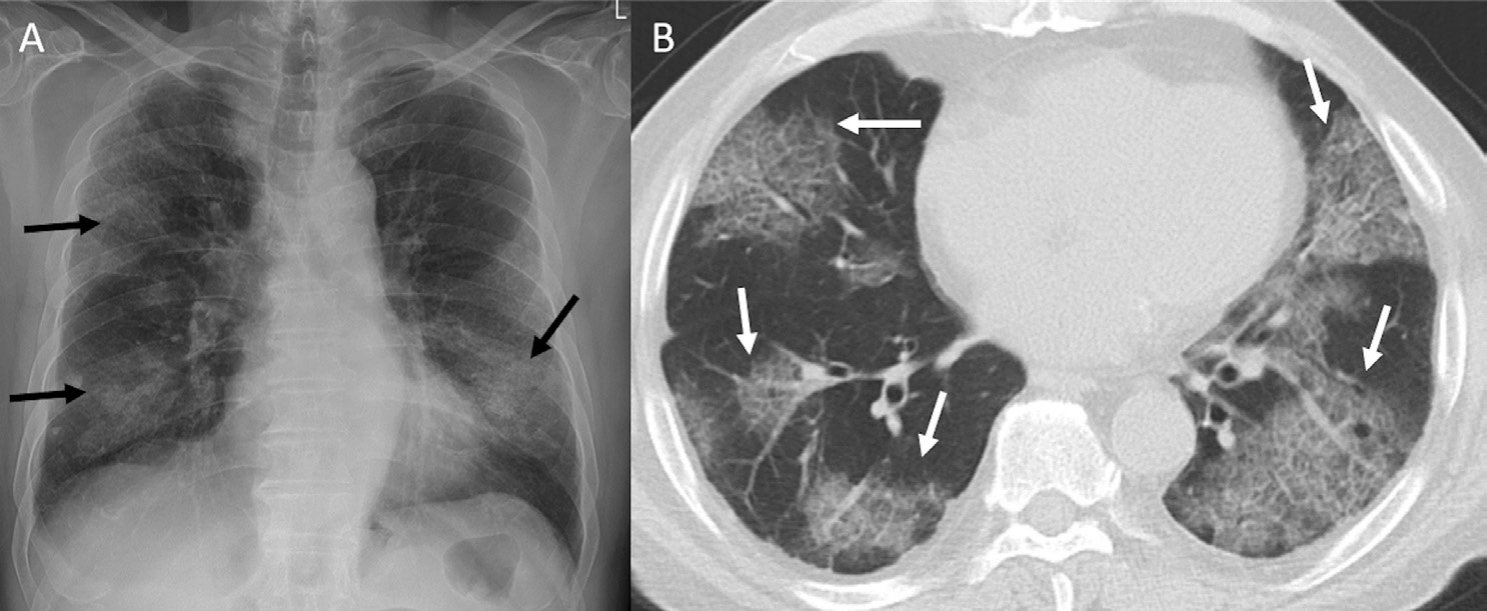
Chest X-ray obtained a week after diagnosis of COVID-19 infection showed multifocal, peripheral bilateral disease. CT obtained on the same day of the radiograph showed peripheral bilateral GGO with superimposed reticular interstiial thickening within the GGO giving a ‘crazy-paving’ appearance. GGO, ground-glassopacity.
